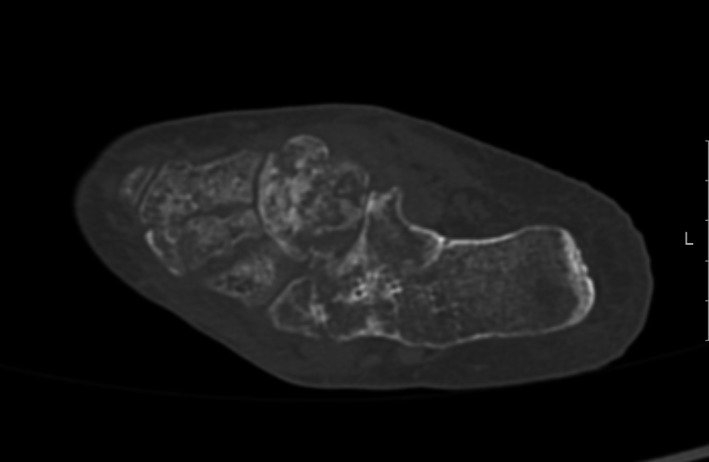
Foot CT revealed osteitis of calcaneus, cuboid, and navicular bone.
